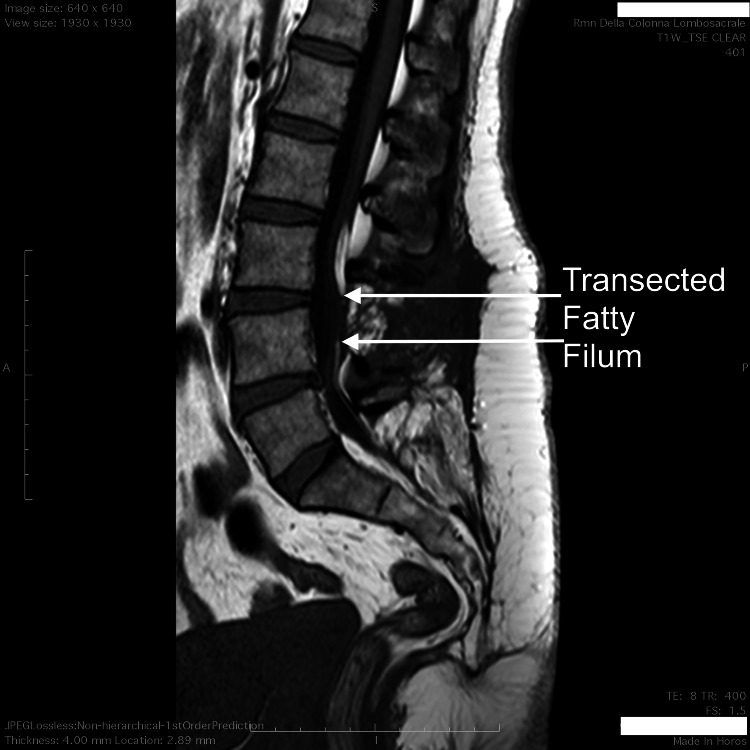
Lumbar MRI after section of the fatty filum. The conus medullaris does not ascend after fatty filum sectioning, but the patient becomes asymptomatic.
